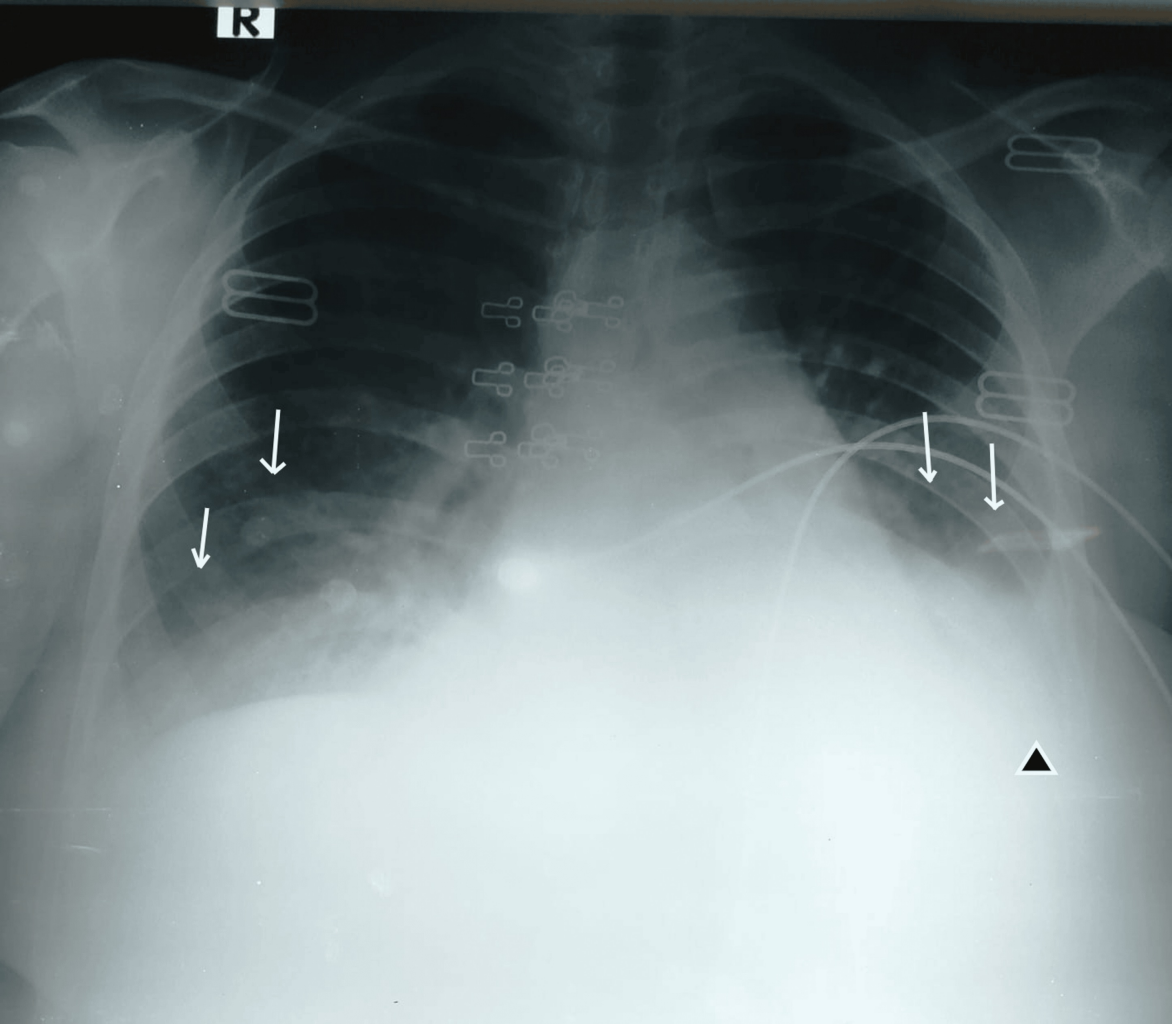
Chest X-ray (Day 2) with arrows showing worsening of bilateral alveolar and interstitial opacities. The arrow head shows left-sided pleural effusion with obliteration of costophrenic angle.
